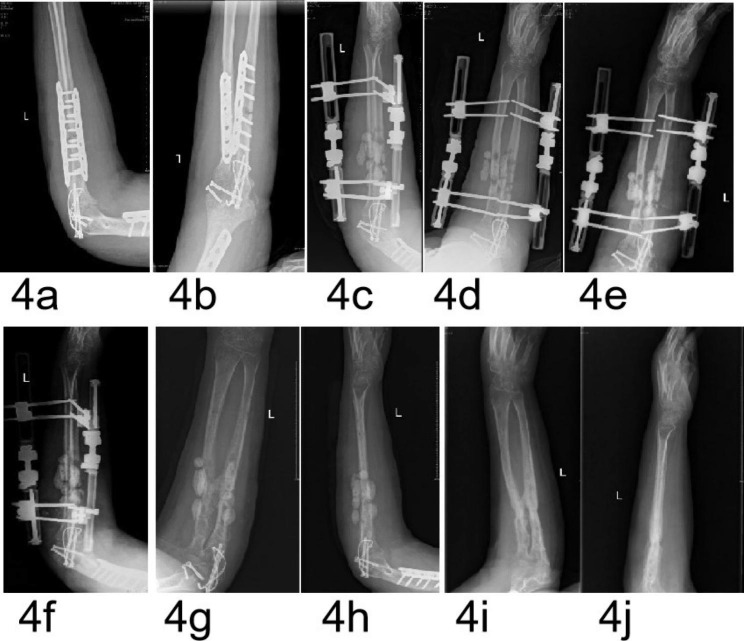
A 44-year-old female patient with a grade IV IMSO. (a,b) AP and lateral radiographs showing bone resorption caused by infection after internal fixation of ulnar and radial fractures. (c,d) AP and lateral radiographs showing the cement beads of PMMA inserted in the defects after debridement. (e,f) AP and lateral radiographs showing minimal new bone formation at three months after insertion of the PMMA. (g,h) AP and lateral radiographs showing continuous new bone formation at four months after insertion of the PMMA. (i,j) AP and lateral radiographs showing clinical healing of the fractures and defects at six months
